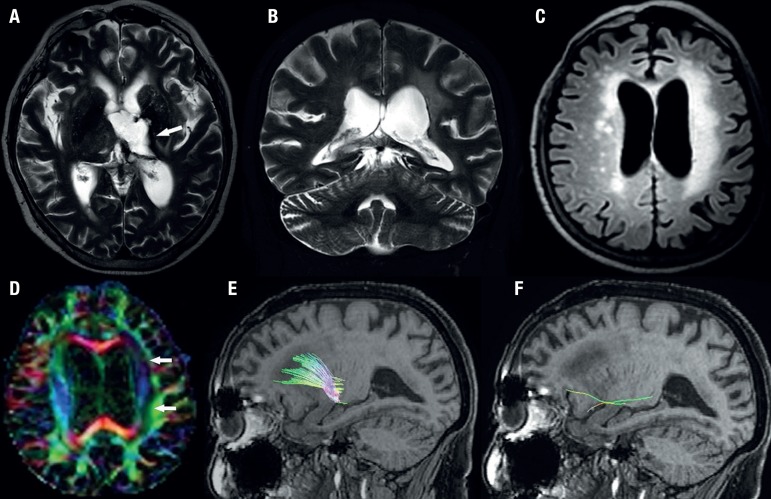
Axial T2-weighted image (A) shows a residual cavity related to
